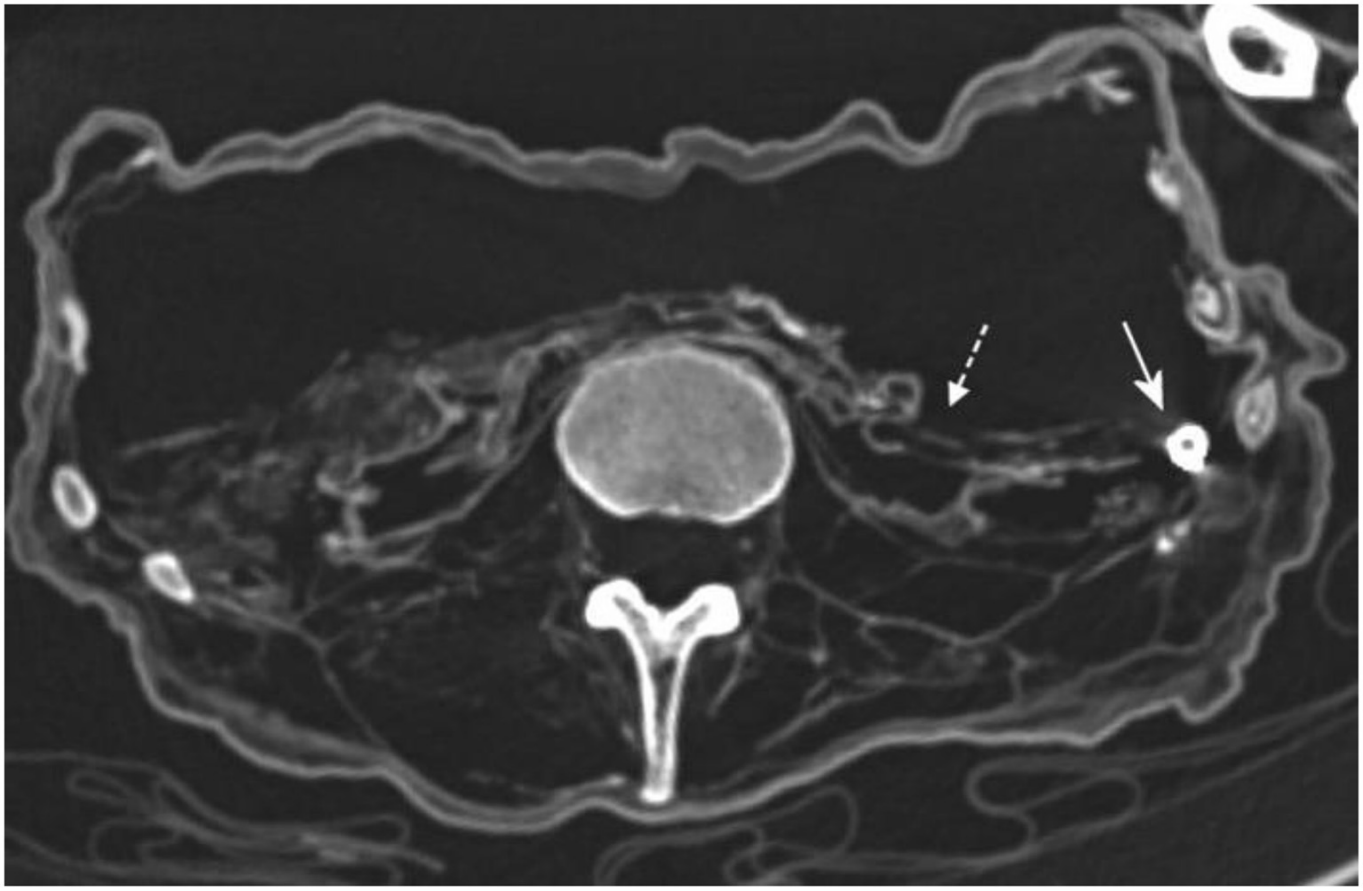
Axial CT reconstruction of the pelvis. On the left side the round foreign material was clearly seen (arrow) as a radiodense sphere with an empty center. This was embedded into lamellar foreign material and possible remnants of intestinal tissue which was seen on both sides of the pelvic cavity (dotted arrow) (image: S. Panzer).
